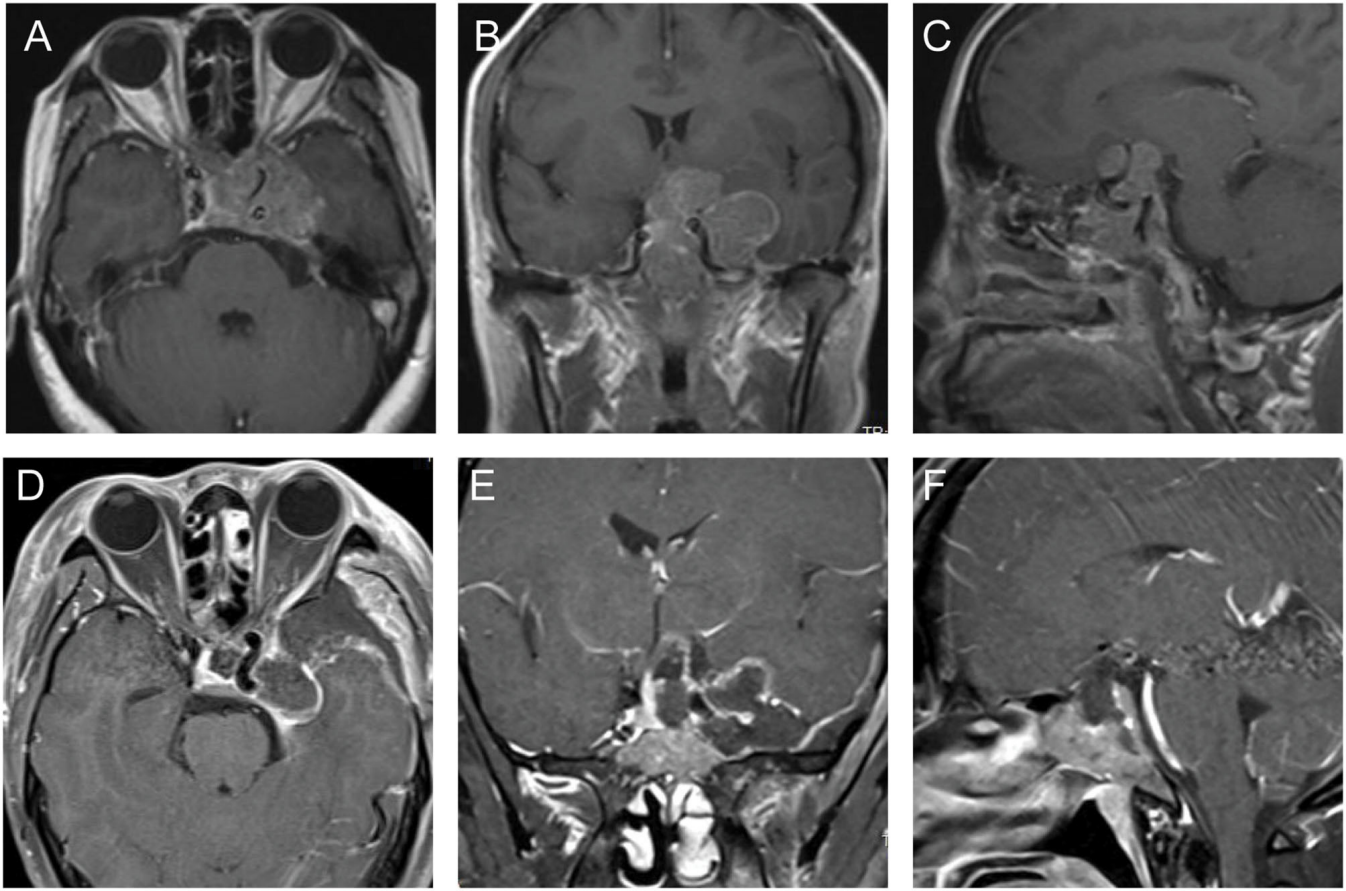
The preoperative and postoperative MRI imaging of case 3. (A–C) Preoperative MRI showed the tumor involved intrasellar, suprasellar region, sphenoid sinus, and the left CS. The intracavernous ICA was completely encapsulated by this tumor. (D–F) Postoperative MRI showed the most of the tumor was resected and part of the tumor remained in the sphenoid sinus.
